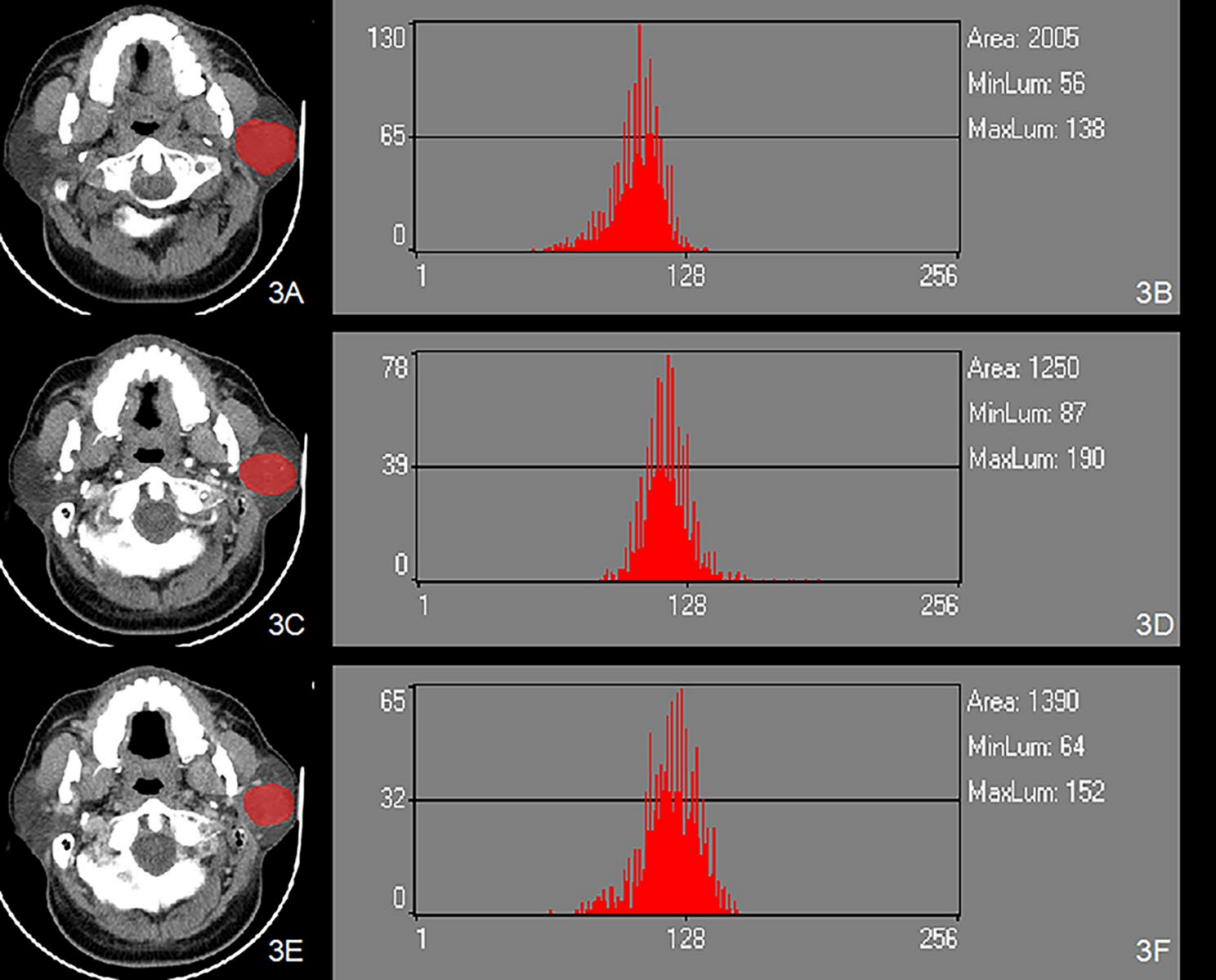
Left adenolymphoma (A) ROI in FSP of Enhanced CT; (B) The histogram of ROI in FSP of Enhanced CT; (C) ROI in AP of Enhanced CT; (D) The histogram of ROI in AP of Enhanced CT; (E) ROI in P of Enhanced CT ; (F) The histogram of ROI in VP of Enhanced CT
